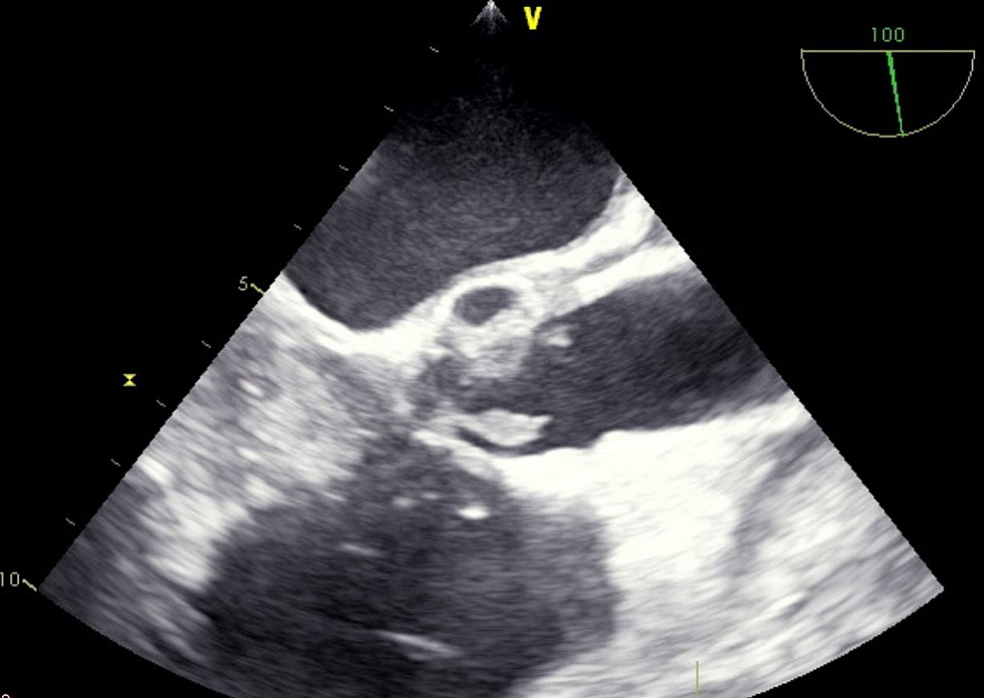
Transesophageal image at the mid-esophageal aortic valve long-axis view demonstrating diffuse bulky vegetations.
